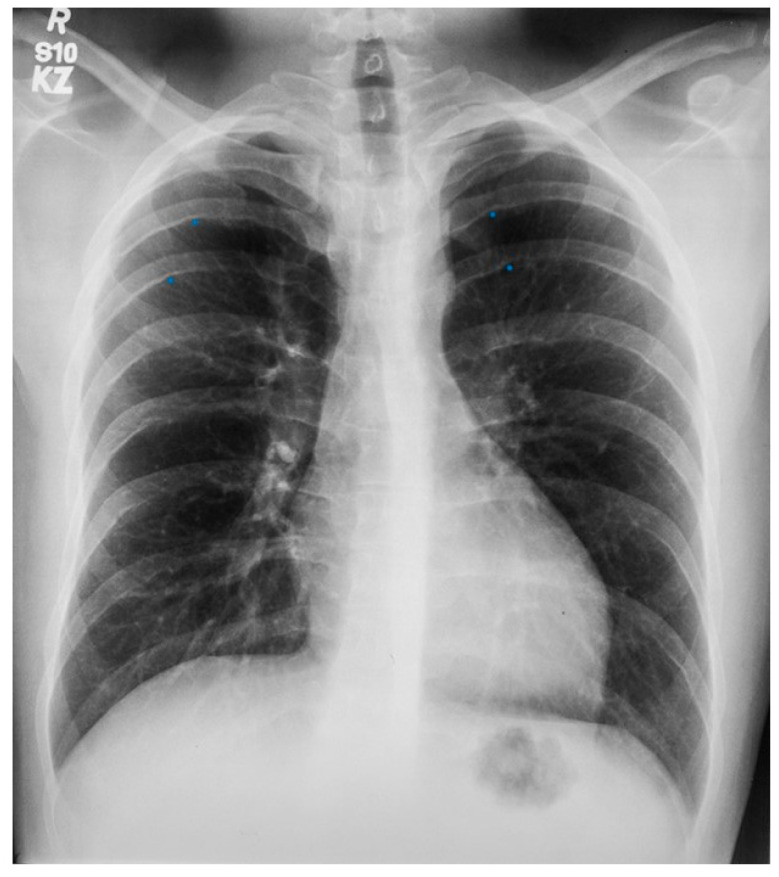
Posteroanterior chest X-ray of coarctation of the aorta: This is the chest X-ray of a 21-year-old male college student who presented for evaluation of hypertension. He spent most of his time studying, because he did not excel in sports due to cramping in his legs when he would run. The blood pressure was 170/80 mmHg in the right upper extremity and there was a marked brachial artery–femoral artery delay when simultaneously palpated. There was a grade 2–3/6 decrescendo diastolic murmur of aortic regurgitation. In the left upper posterior chest there was a long systolic murmur across the coarctation. This chest X-ray is well centered and there is a good inspiration. The cardiothoracic ratio was at the upper limits of normal and there is straightening of the left heart border, due to left ventricular volume overload from severe aortic regurgitation and left ventricular hypertrophy from his coarctation. There is rib notching of the undersides of ribs 4–8 bilaterally (indicated by four blue dots) from enlarged and coiled intercostal arteries carrying collateral blood flow. There is no three-sign, perhaps because this is not an anteroposterior film. He went on to have surgical repair of the coarctation via left lateral thoracotomy and then aortic valve replacement via median sternotomy for a bicuspid aortic valve with severe regurgitation. His blood pressure and his heart size both decreased and his tolerance for exertion improved.
